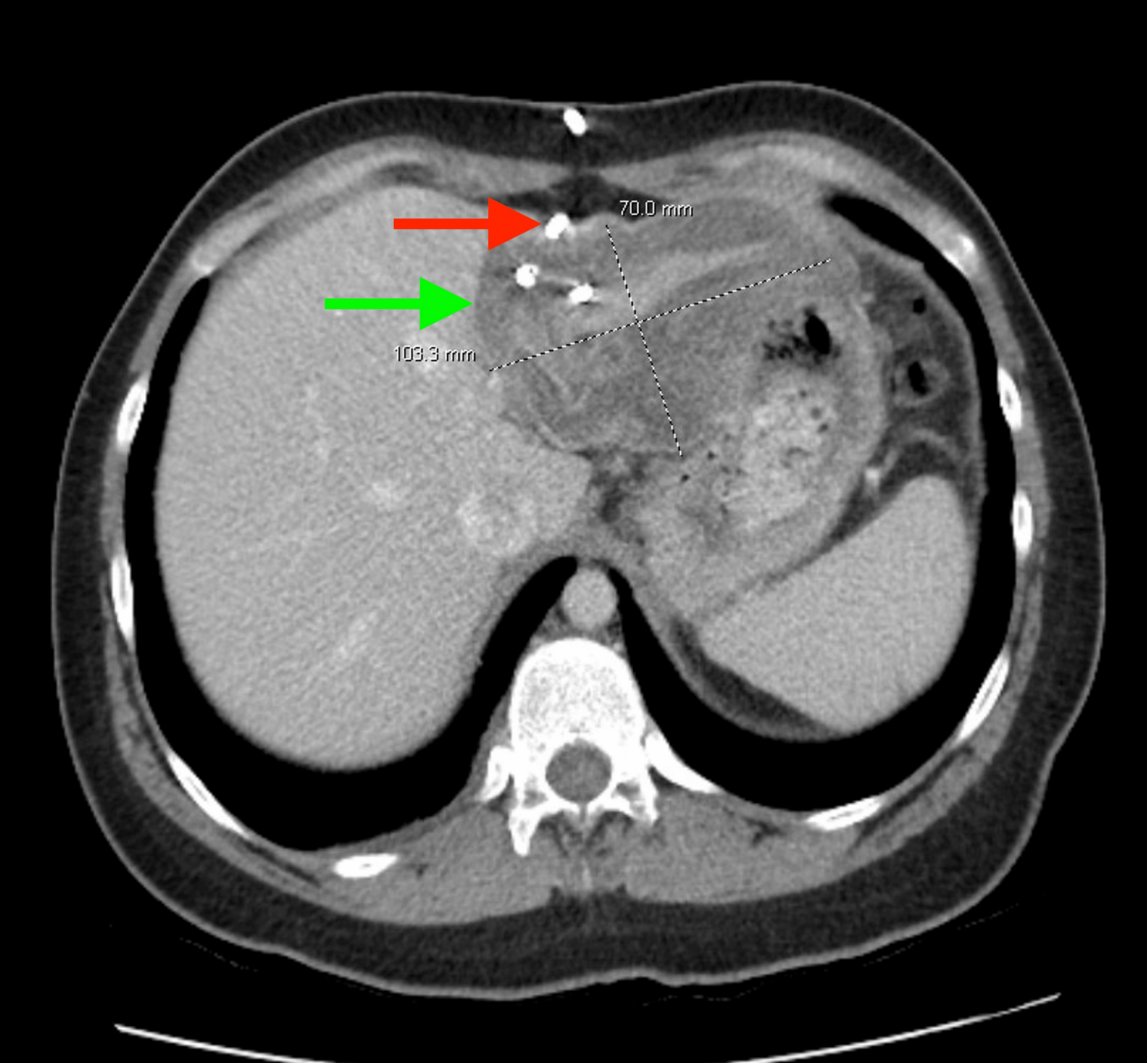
CT abdomen and pelvis status after percutaneous drainage of hepatic cyst CT abdomen and pelvis status post percutaneous drainage of hepatic cyst, showing an interval decrease in size of the collection in the left lobe of liver (green arrow), with a pigtail drainage catheter (red arrow) seen within the collection. The collection had decreased from the prior measurement of 11.8x8.8 cm to a 10x7 cm.
